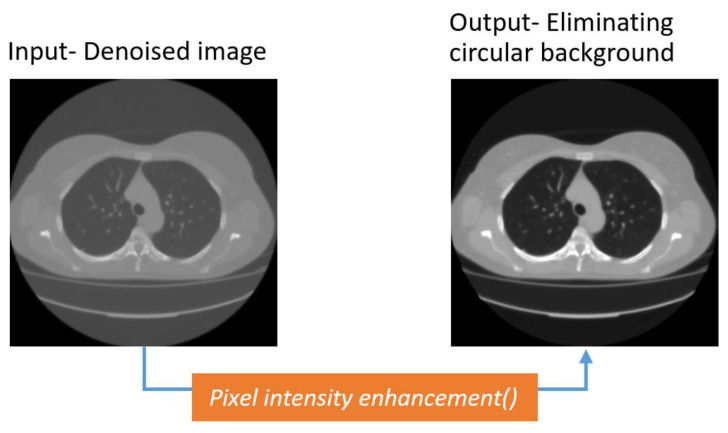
Enhanced CT scan with defocused circular background.
